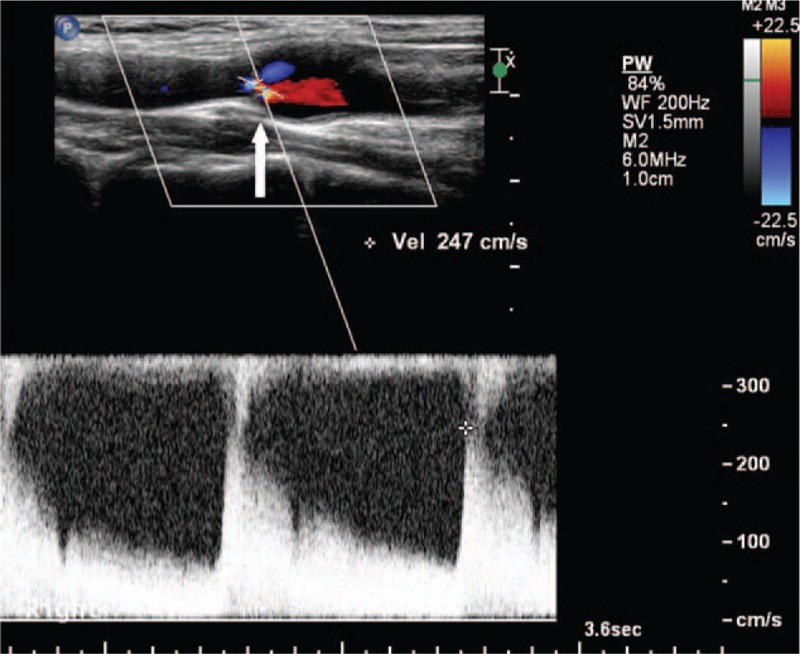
Color Doppler flow imaging (CDFI) showed turbulent color jet through this lumen of high velocity blood flow of 247 cm/s (arrow). CDFI = Color Doppler flow imaging.
